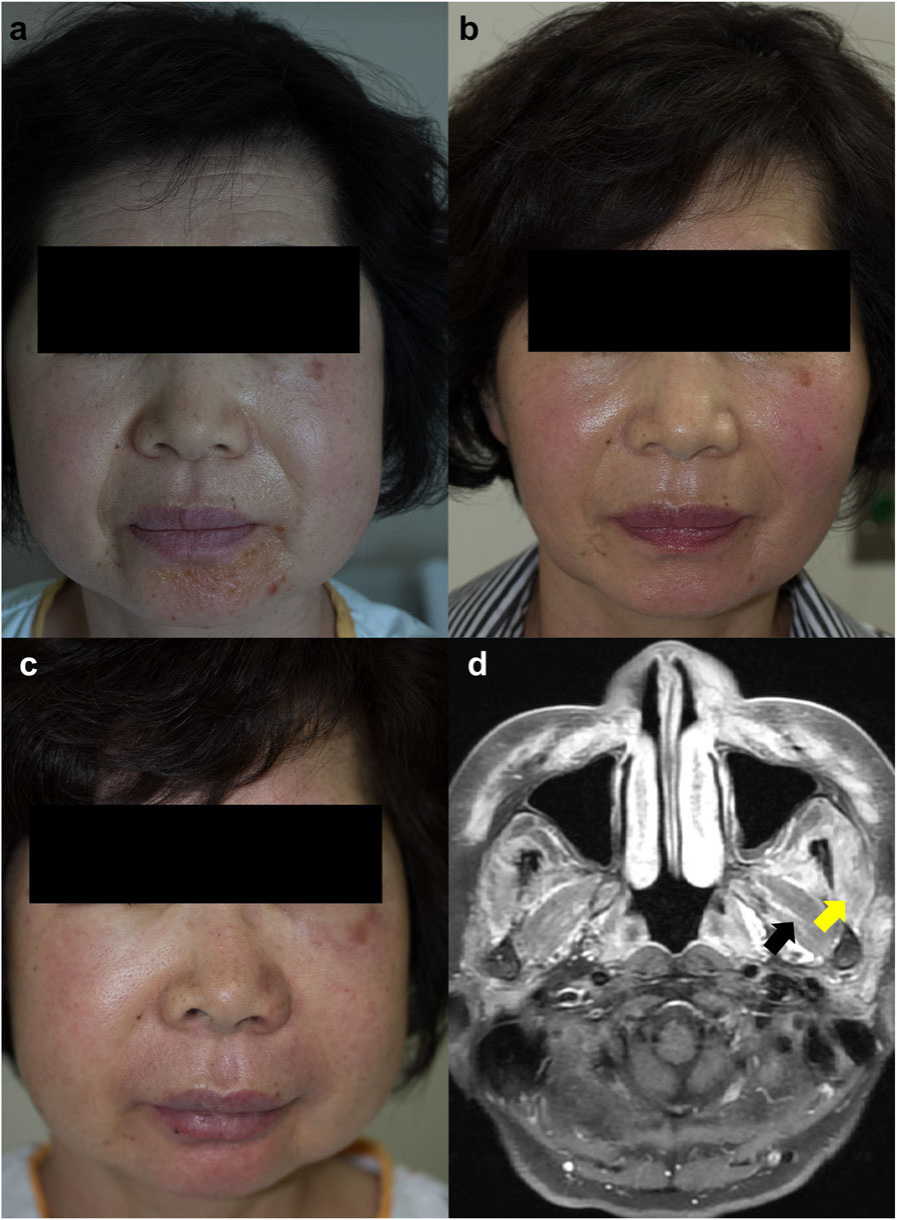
Isolated facial edema and subclinical facial muscle involvement. At initial presentation there was bilateral swelling of the face and of the left eyelid, accompanied by eczematoid eruption on the chin (a). After approximately two weeks of medium-dose glucocorticoid therapy the edema and the lesions were completely resolved (b). However, combination therapy with either azathioprine, mycophenolate, or methotrexate was not successful in maintaining remission at a prednisolone dose lower than 15 mg per day. At the last relapse, there was marked aggravation of facial and eyelid edema (c). Gadolinium-enhanced MRI scan of the head shows diffuse edematous change with enhancement of bilateral masticator muscles (d, masseter muscle [yellow arrow] and lateral pterygoid muscle [black arrow]). There was also swelling of bilateral extra-ocular muscles and edematous change with fat infiltration in the subcutaneous tissues of the anterior scalp, periorbital area, buccal space, and anterior cervical fascia (not shown)
